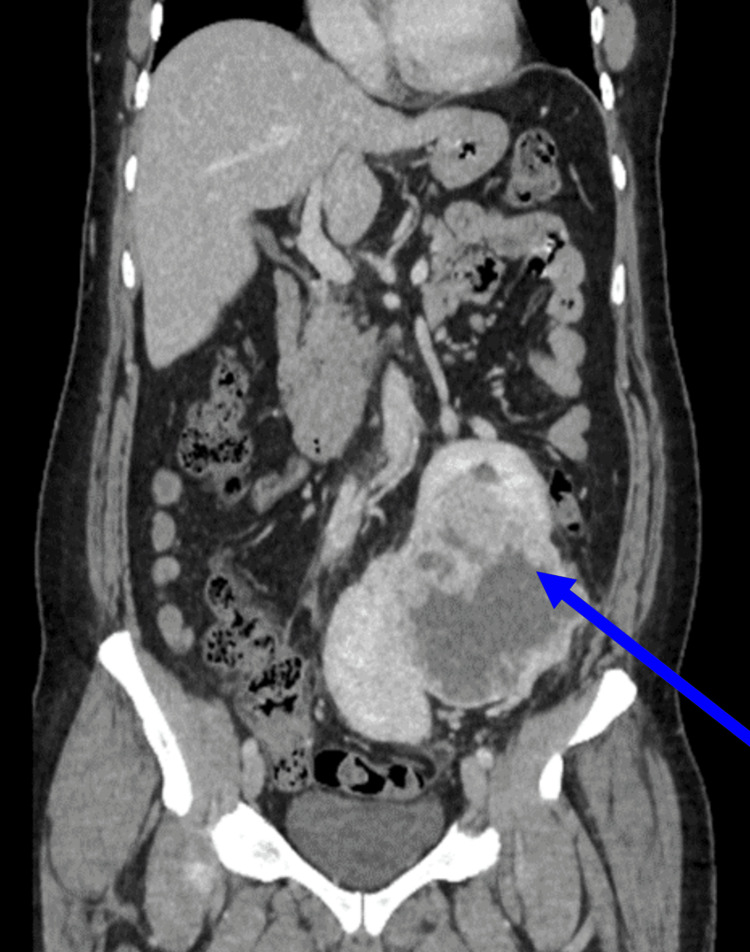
CT abdomen pelvis with a contrast coronal view, showing an empty right renal fossa and an ectopic right kidney fused to the lower pole of the left kidney CT: computed tomography
